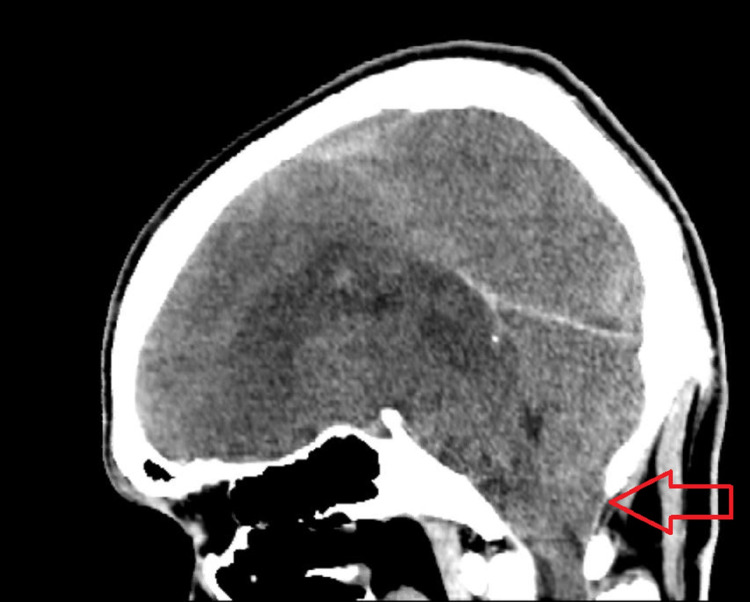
Computed tomography, sagittal section, showing evidence of cerebellar tonsillar herniation through foramen magnum. (Red arrow showing the level of the foramen magnum.)
